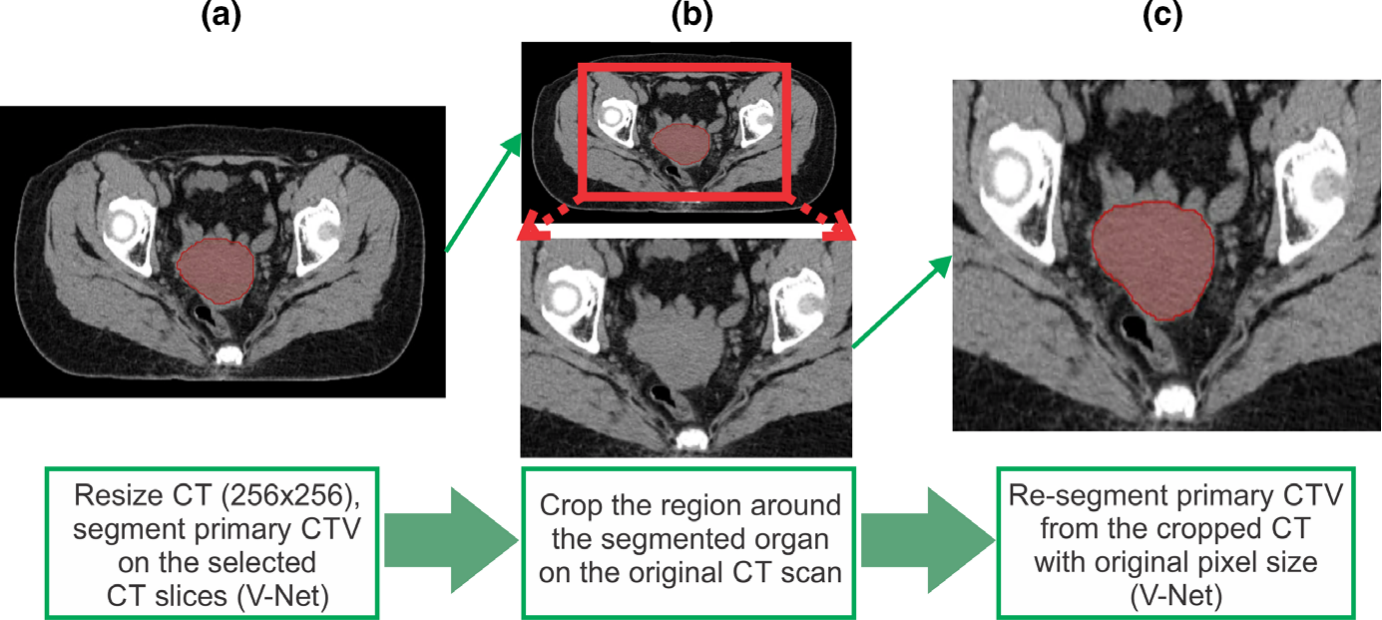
Segmentation using cropped three‐dimensional images for better accuracy. (a) Resize the computed tomography (CT) from 512 × 512 to 256 × 256 pixels and then segment the organ of interest and find the center of mass, (b) crop the region around the segmented organ on the original 512 × 512 CT scan, and (c) resegment the organ of interest on the cropped image. [Color figure can be viewed at wileyonlinelibrary.com]
